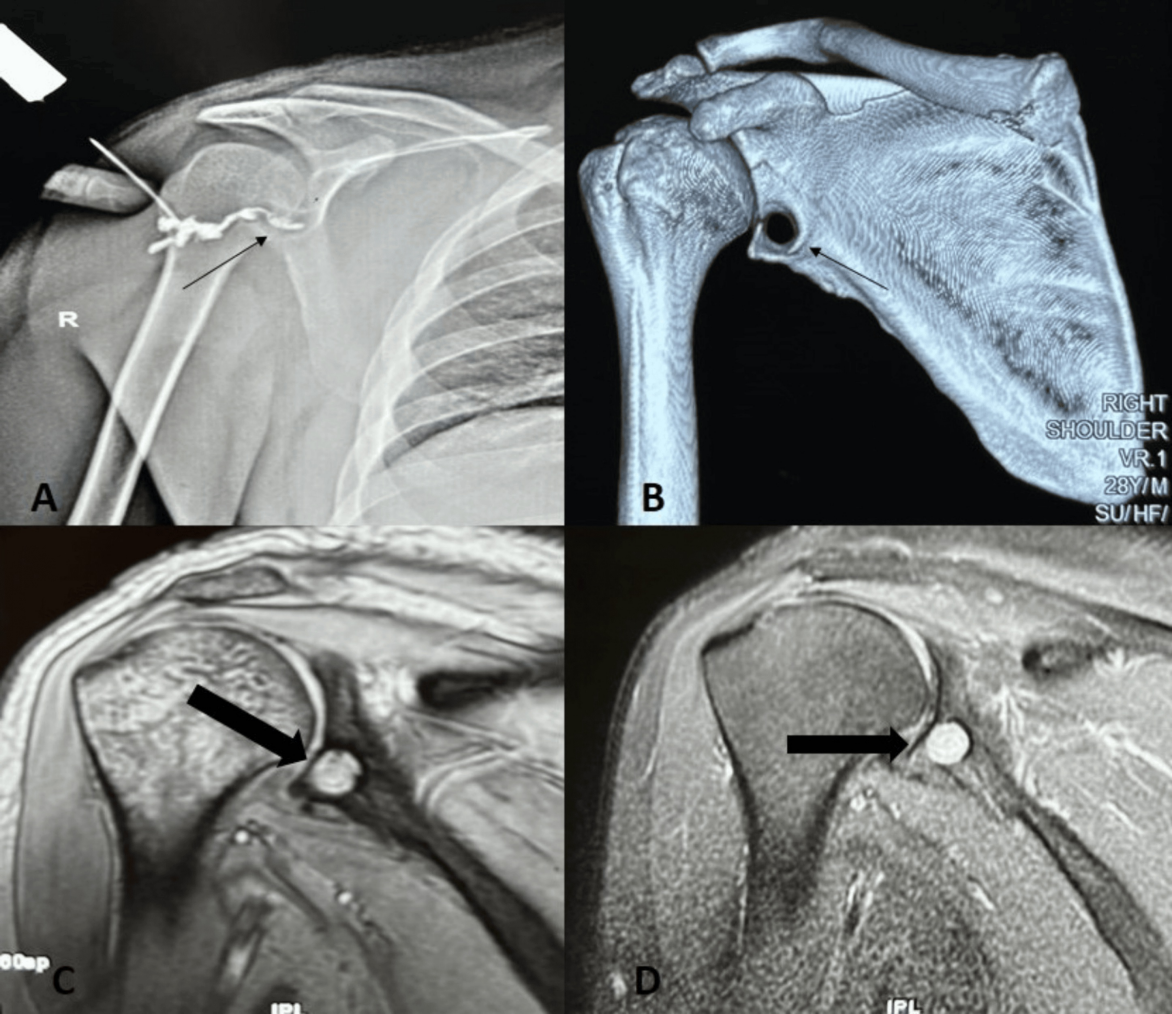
Pre-operative images A: Sinogram showing the sinus tract communicating with the antero-inferior glenoid rim. B: Computed tomography scan showing a well-corticated defect in the antero-inferior glenoid. C: T1-weighted magnetic resonance image showing a hypointense lesion. D: T2-weighted magnetic resonance image showing a hyperintense lesion.
